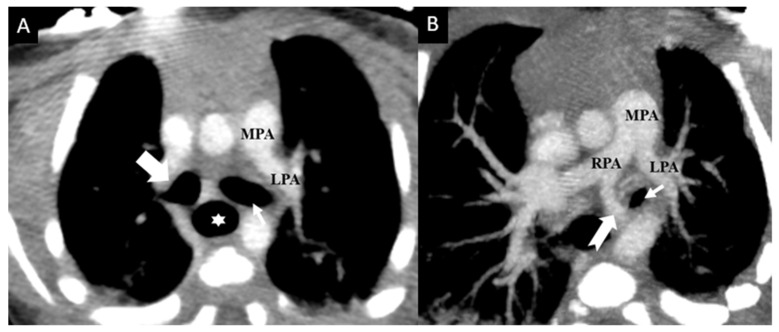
Newborn male with complex congenital heart disease (double outlet right ventricle, ventricular septal defect, and aortic coarctation) with PALPA. Contrast enhanced axial (A) and (B) axial oblique reconstructed Maximum Intensity Projection (MIP) images from chest CT data demonstrate a duplicated left pulmonary artery with a normally arising left upper lobe pulmonary artery (LPA) from the main pulmonary artery (MPA) coursing anterior to the left main bronchus (thin small arrow), while an anomalous left lower lobe pulmonary artery (notched arrow) coursing inferior anterior to the left main bronchus and left lateral to the esophagus (star). No anomalous vessel was seen between the trachea and the esophagus. Right main bronchus is marked with a bold white arrow.
